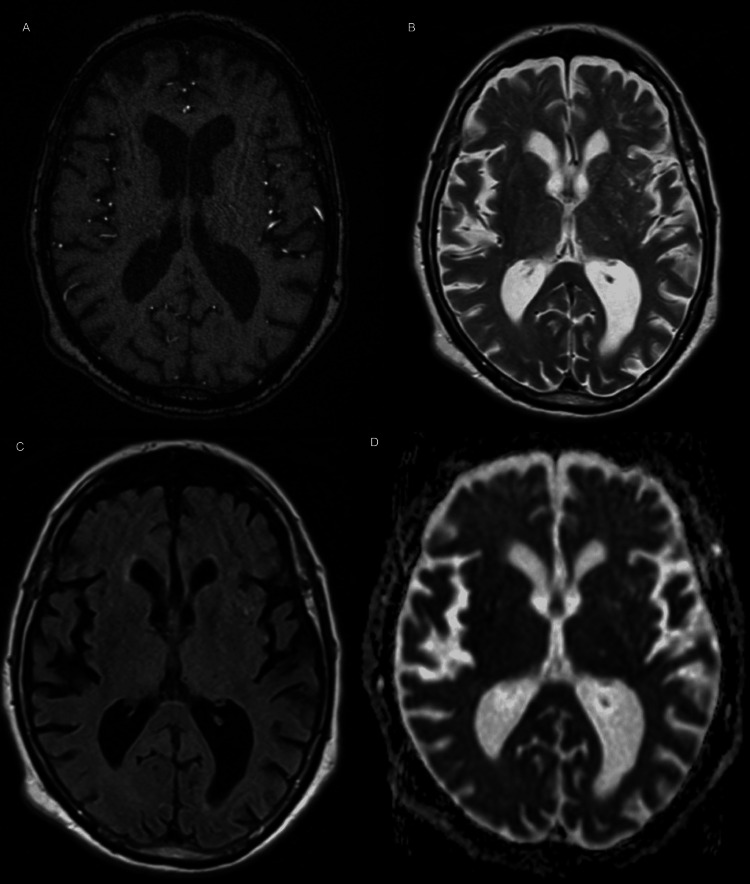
Axial magnetic resonance images: T1-weighted sequence (A), T2-weighted sequence (B), T2-FLAIR (C), and diffusion-weighted image (D), showing no evidence of lesions in the basal ganglia. FLAIR: fluid-attenuated inversion recovery
